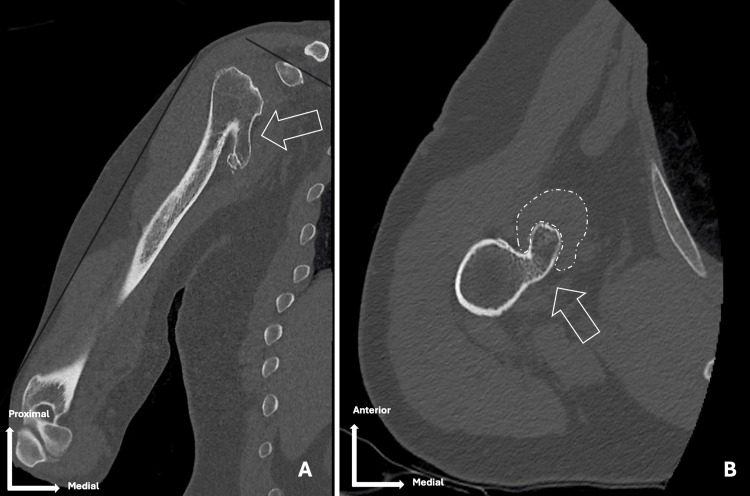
(A) Coronal and (B) axial view CT scan (bone window) White arrows indicate a large proximal humeral osteophyte penetrating the coracobrachialis muscle (white dotted line) near the musculocutaneous nerve CT: computed tomography
